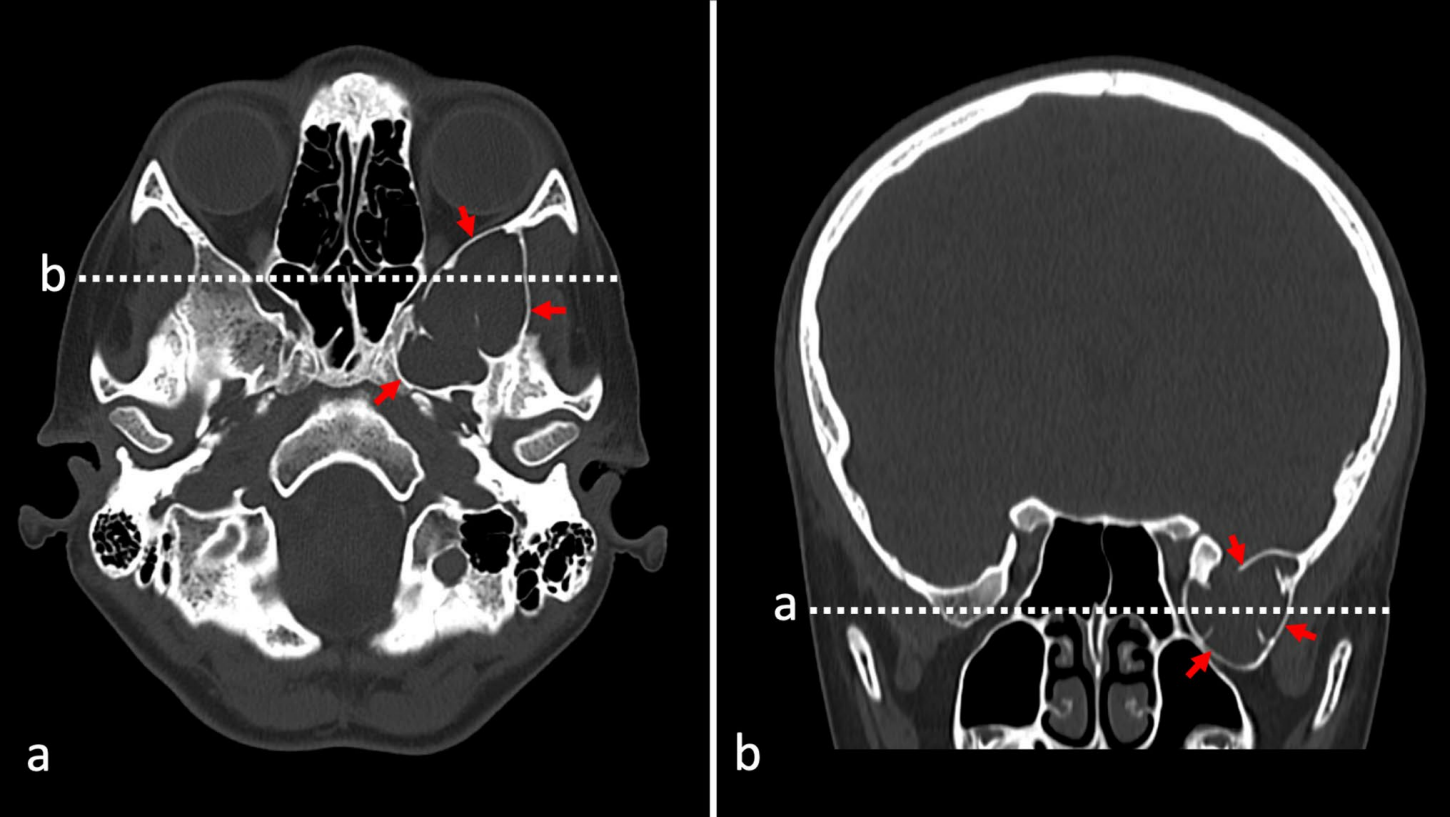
Preoperative non-enhanced head CT scan axial cross-section (a) with white dotted line marking level of the coronal section (b). Cyst is marked with red arrows
